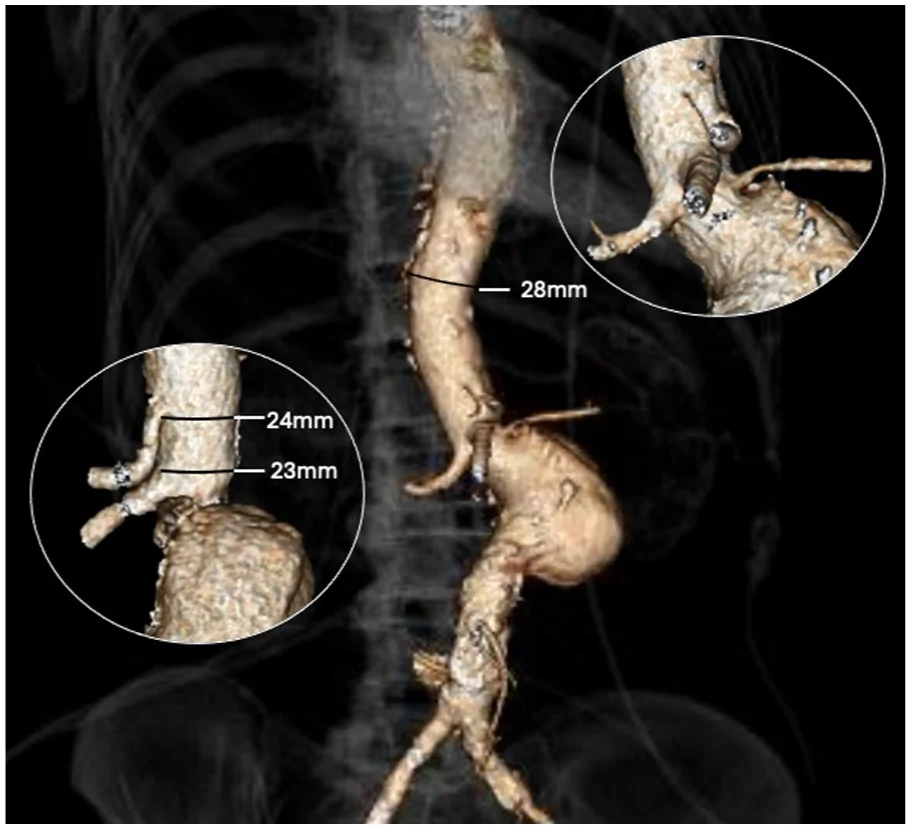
Preoperative 3-dimensional volume rendering computed tomography angiography (3D VR CTA) of Juxtarenal Abdominal Aortic Aneurysm (JAAA). Within circles, the close-up of visceral vessels; intravessel diameter (IVD) at the level of proximal fixation, CT, and SMA.
